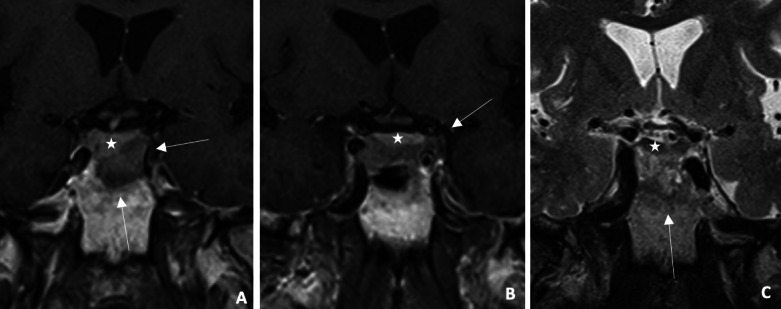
Infiltrative lesion (arrow) centered on the sellar region (A–B) Coronal post‑constrat T1WI: right deviation of pituitary stalk and pituitary gland (star). (C) Coronal T2WI: heterogeneous lesion , adjacent to hypointense pituitary gland. MRI shows infiltrative sellar lesion displacing pituitary stalk/gland.
